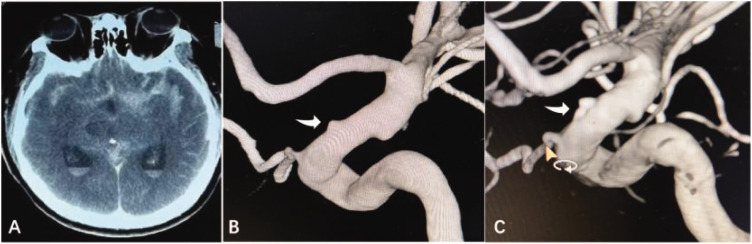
Computed tomography (CT) and digital subtraction angiography (DSA) imaging of a representative case. (a) CT showing subarachnoid hemorrhage (SAH). (b) DSA showing a small blood blister-like aneurysm (BBA; white arrow) of the supraclinoid internal carotid artery (ICA) and (c) BBA was treated using stent-assisted coil embolization (white arrow).
